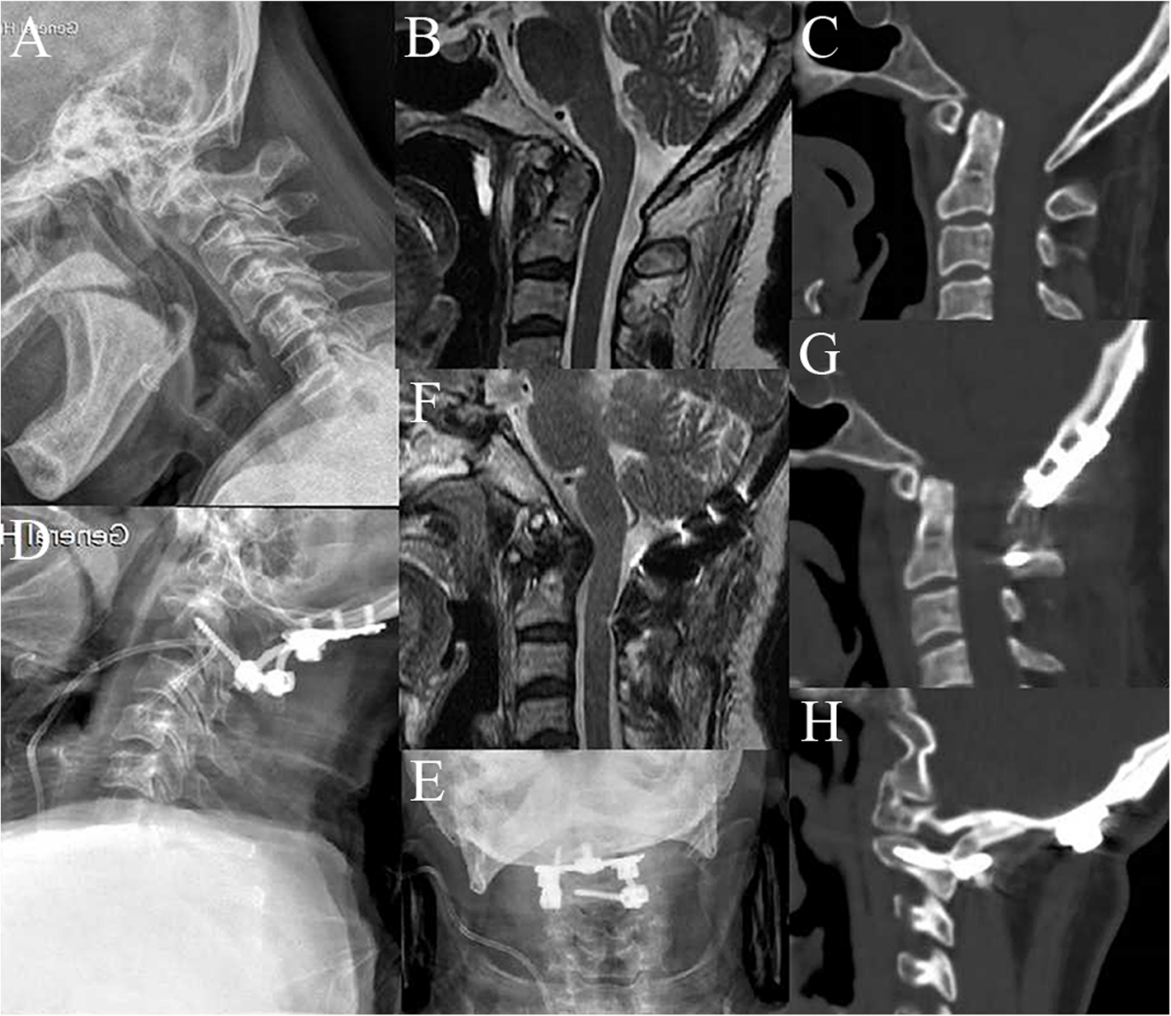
A 62-year-old man, diagnosed with basilar invagination with atlantoaxial dislocation, underwent an OF surgery. Preoperative images of cervical lateral radiographs (a), sagittal CT scan (b), and sagittal MRI (c) showed evidence of basilar invagination with atlantoaxial dislocation and compression on the ventral. Cervical lateral radiographs (d, e) after an OF operation showed satisfactory location of internal fixation. Postoperative sagittal MRI (f) and sagittal CT scan (g) showed repositioning of atlantoaxial dislocation and alleviation of compression on the medulla. CT image (h) from the 6-month follow-up showed solid bone fusion
